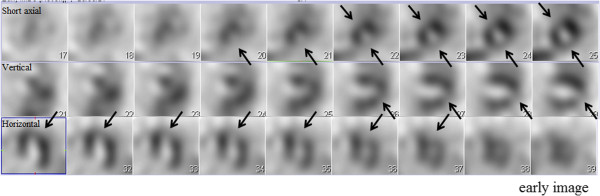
I-123 metaiodobenzylguanidine scintigram recorded 20 days after the cardiac event. Decreased uptake of I-123 metaiodobenzylguanidine was observed in the inferolateral and anteroseptal walls of the left ventricle (arrows).
